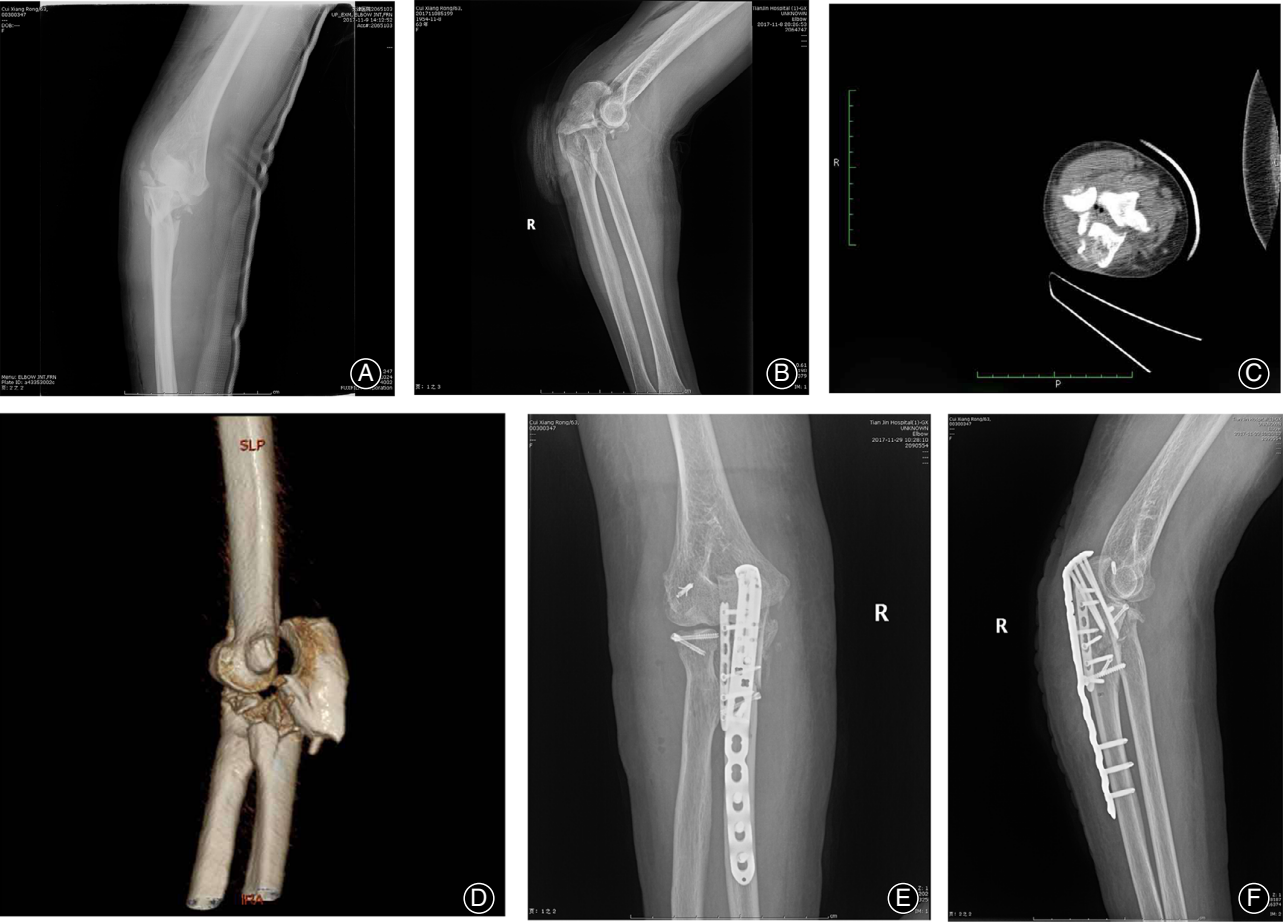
A 63‐year‐old woman falling from a standing height with the right dominant elbow injured. (A, B) Initial anteroposterior and lateral radiograph showed proximal ulna fracture and radial head fracture, accompanied by comminuted metaphyseal fracture and radiocapitellar dislocation. (C, D) A computed tomography image showed the PRUJ dislocation. (E, F) The anteroposterior and lateral radiograph obtained following the operative treatment.
